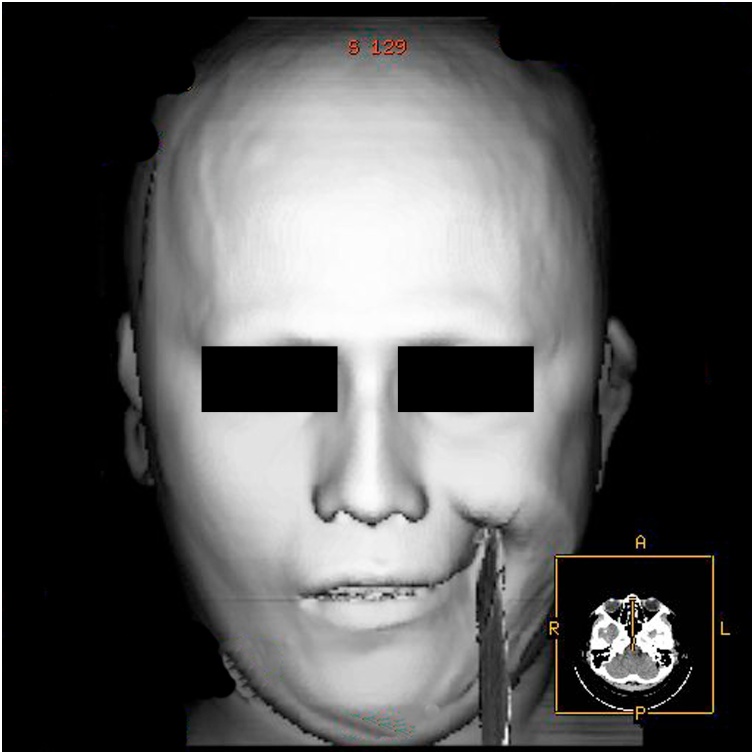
Preoperative 3D head CT reconstruction showed foreign the foreign wooden body penetrated the man's left nasolabial fold.
